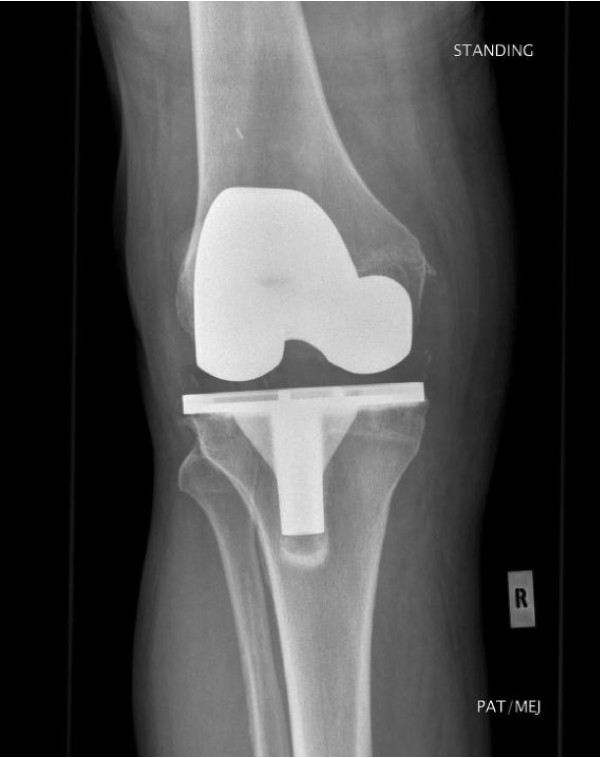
Radiograph post-medial femoral osteophyte excision (arrow). Tibial and femoral components are unchanged.
